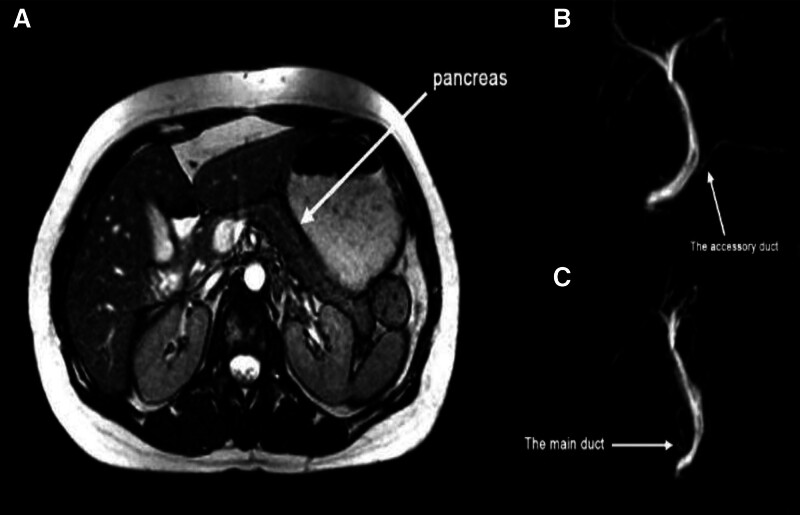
transverse section MRI (A) showed a normal pancreas (arrow), MRCP of pancreatic duct (B) showed the accessory duct have a common outlet with the collecting duct (arrow), MRCP of pancreatic duct (C) showed the main pancreatic duct have a separate outlet above the outlet of the collecting duct (arrow). MRCP = magnetic resonance cholangiopancreatography, MRI = magnetic resonance imaging.
